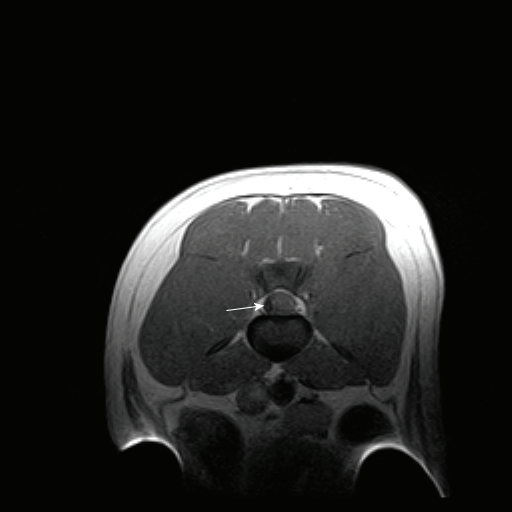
Magnetic resonance imaging of the lumbar spinal cord, case 4. Transverse T1 weighted images of the spinal cord at the level of vertebrae L5 revealing a hypointense area (arrow) lateralised to the right and compressing the spinal cord towards the left.
